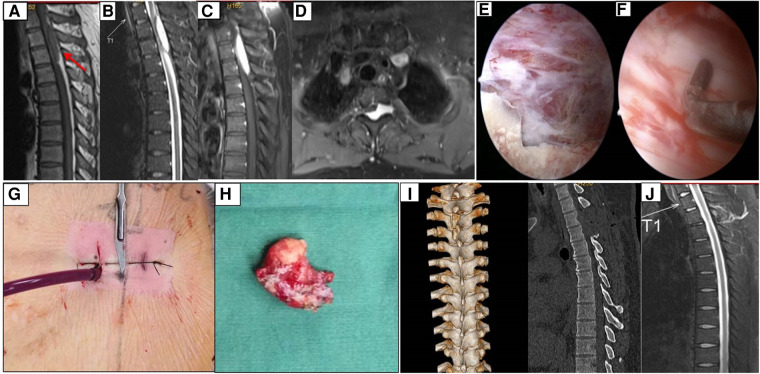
Case 1, extradural hemangioma was en bloc removed by unilateral biportal endoscopy. (A–C) The mass was hypo-intensity on T1, hyper-intensity on T2, and homogenous enhancement after Gd administration. (D) The axial image showed the mass involved the left foraminal, crossed the midline, and spinal cord was obviously compressed. (E,F) Endoscopic findings revealed a reddish mass compress the dural sac, and the dural sac was intact after totally removal of the mass. (G,H) The incisions and the mass resected. (I) Reconstructed CT showed hemi-laminectomy from T2 to T3, and the fact joint and spinal process was reserved. (J) MRI showed the spinal cord was decompressed and no recurrence was observed after six months follow-up.
